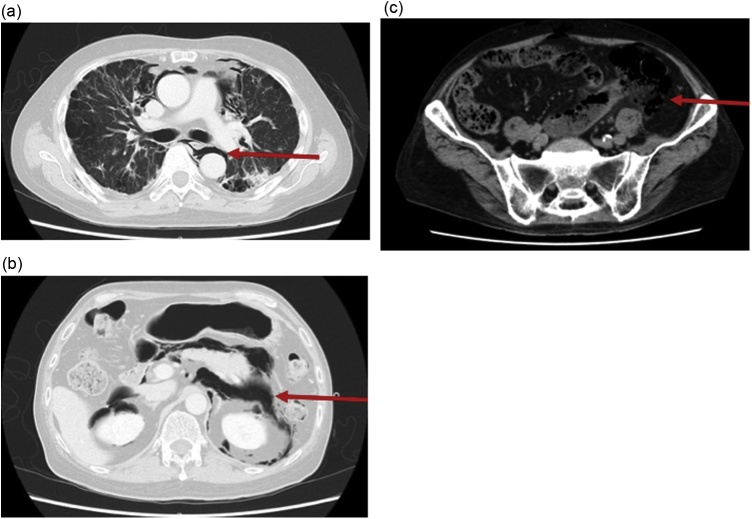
Selected images from a computed tomography (CT) scan of the chest, abdomen, and pelvis (a, b, and c: axial images; d: reformatted coronal images). CT demonstrated perforation of the sigmoid colon into the mesentery (c, red arrows). Using lung windowing, gas was found extensively, from the retroperitoneum to the mediastinum (a, b, and d, red arrows).
